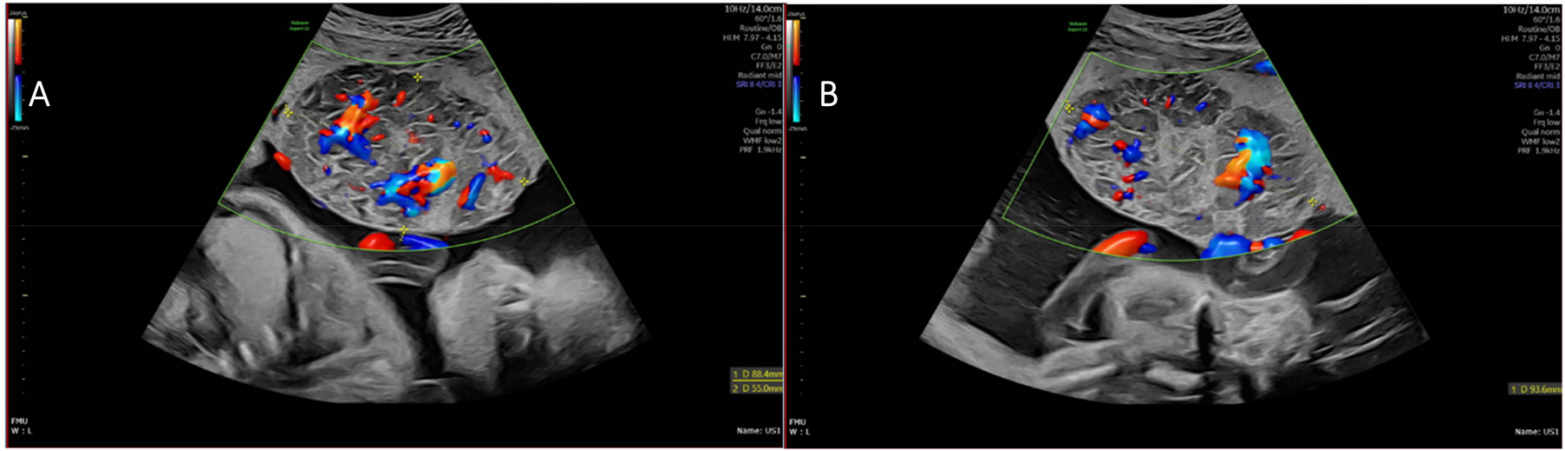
(A and B) Large placental chorioangioma visualised on ultrasound measuring 9.4×8.8×5.5 cm in two dimensions. Colour flow Doppler revealing a large central artery located close to the umbilical cord insertion.
